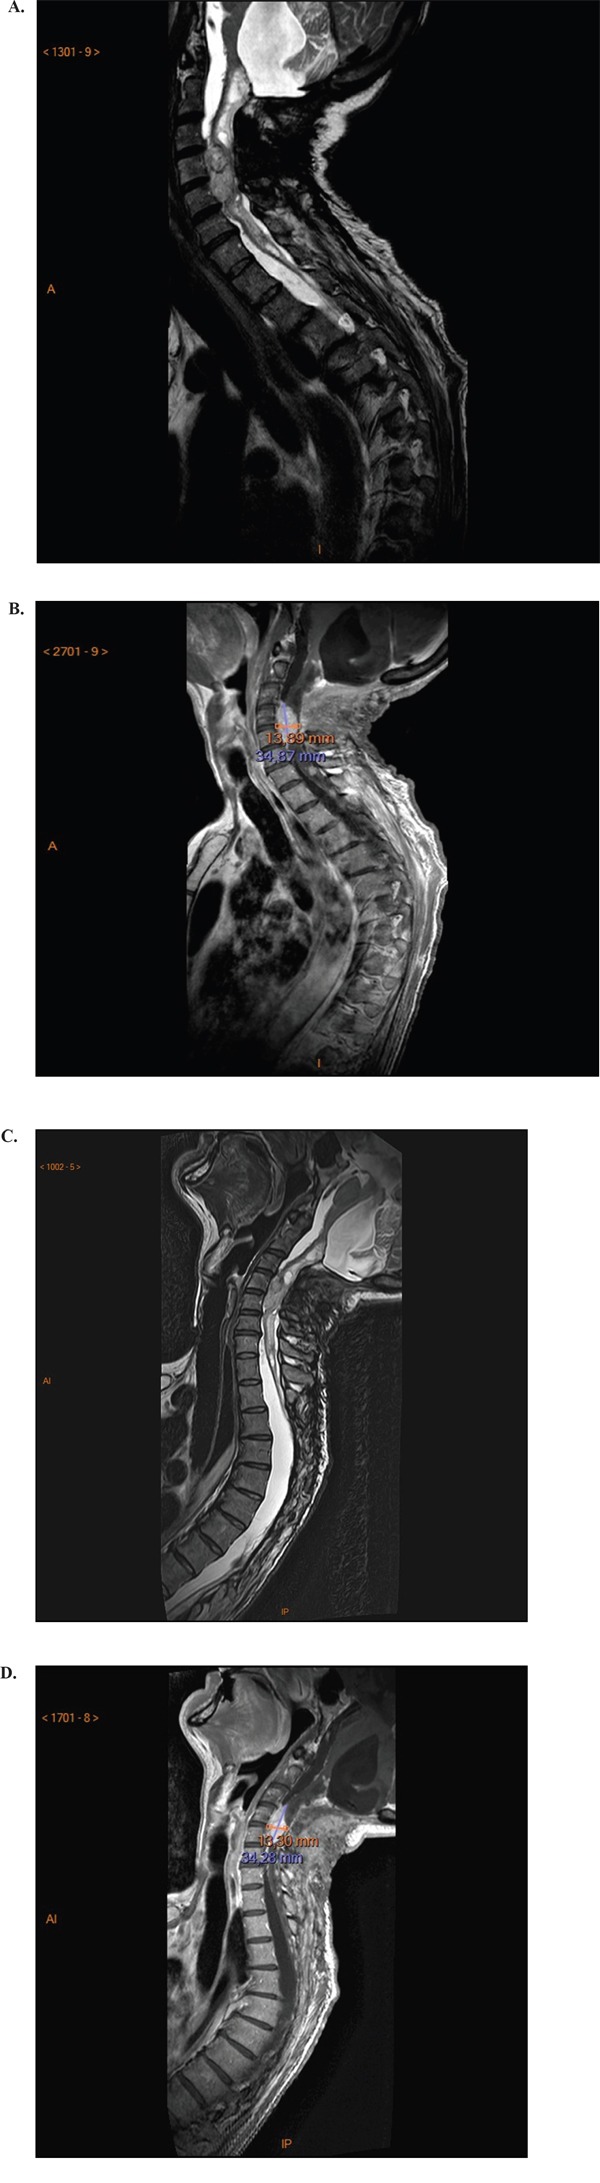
Magnetic resonance imaging showing a large hemangioblastoma at the spine cervical level at baseline (A & B); and no change in hemangioblastoma size after 4 weeks’ sunitinib (C & D).
